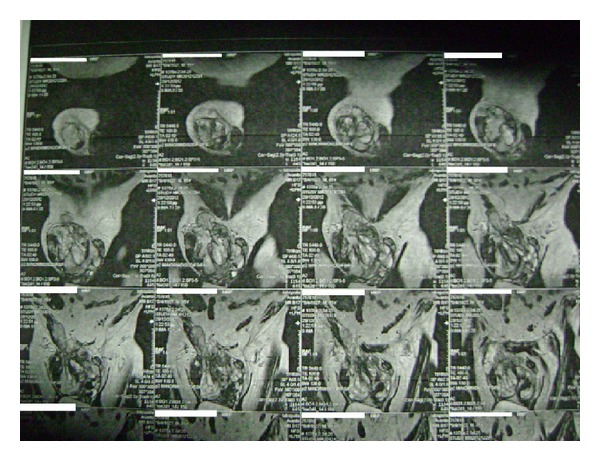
MRI of the pelvic depicting the paratesticular mass.
